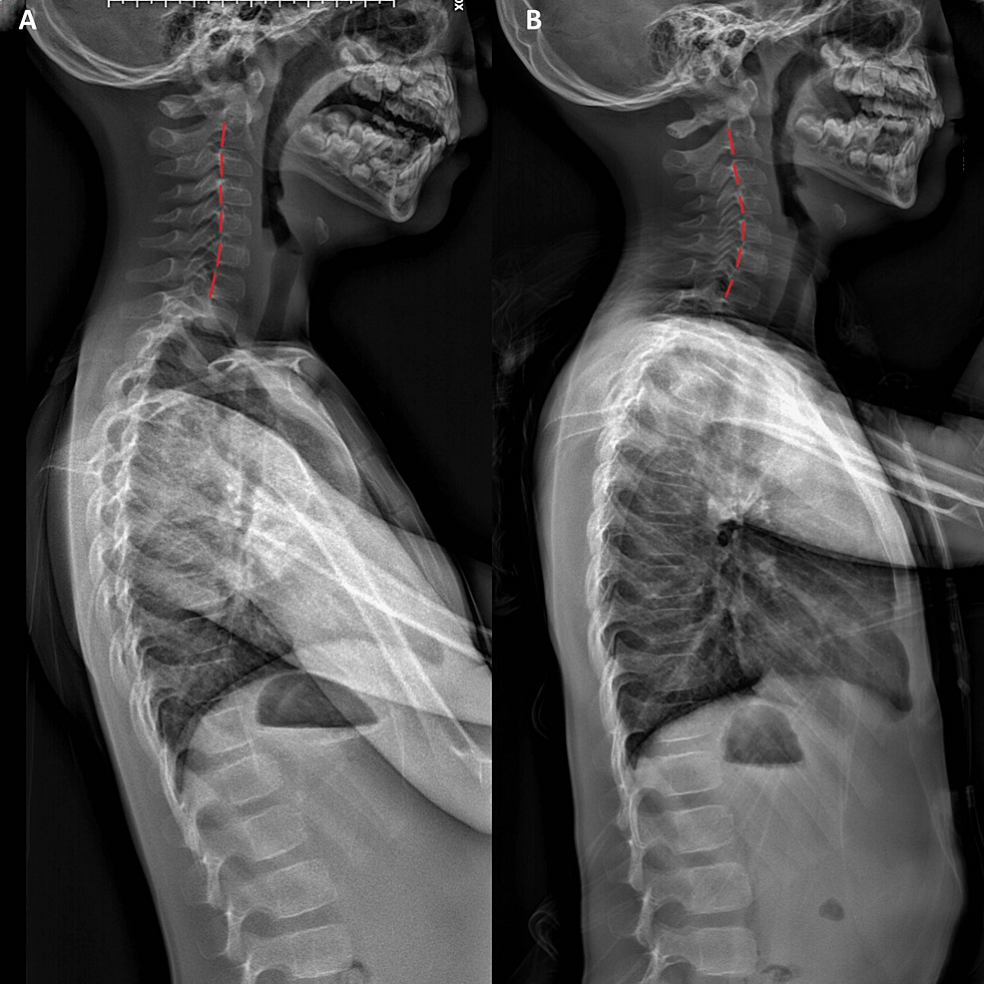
EOS® (EOS Imaging, Paris, France) full-spine radiography in sagittal view. (A) Radiographic imaging of the cervical spine revealed a reduction in cervical lordosis (red line) before treatment. (B) At the nine-month follow-up, the patient’s cervical range of motion was improved bilaterally and cervical lordosis was restored (red line).
